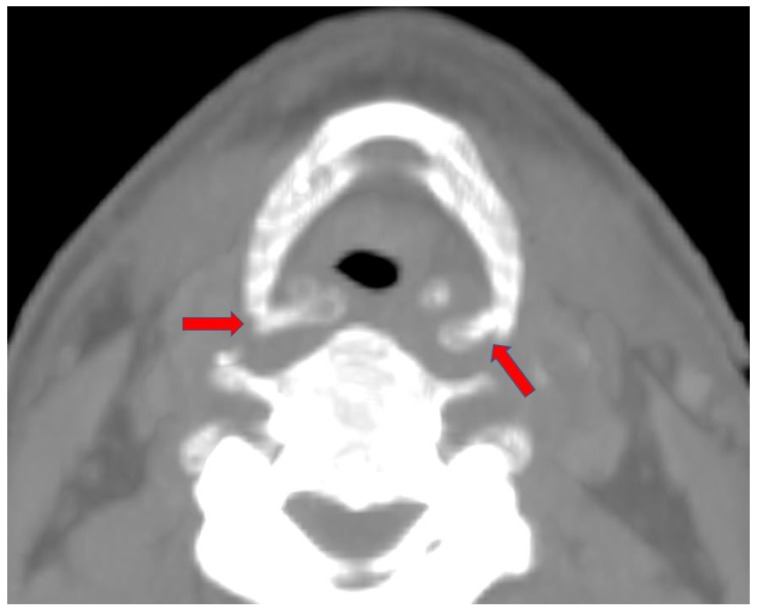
An axial MIP CT image demonstrates the bilateral fracture of the greater horn of the hyoid bone (red arrows).
